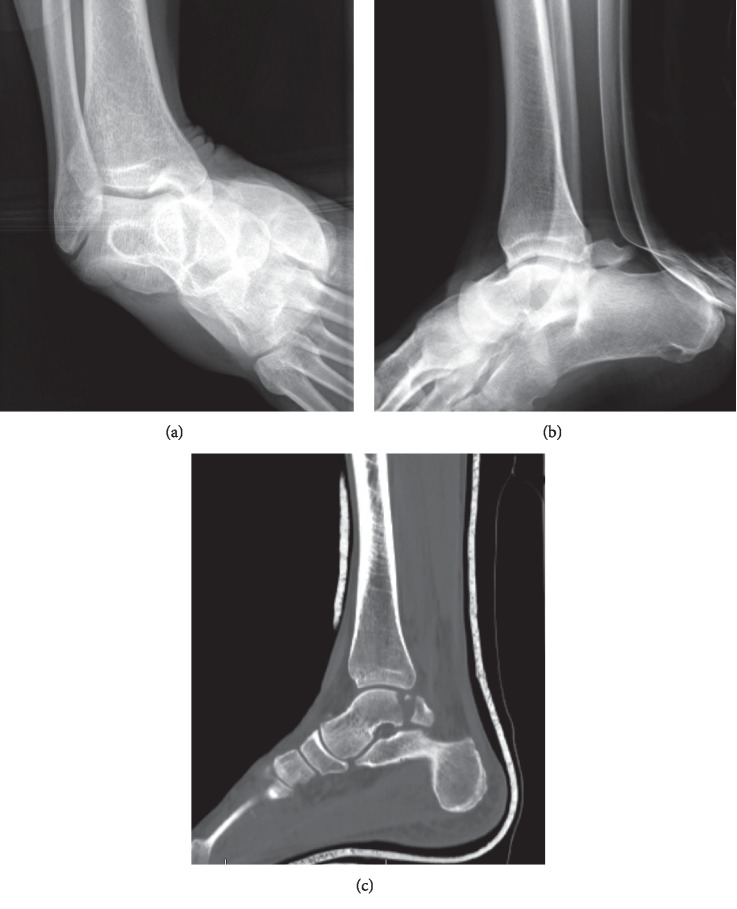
AP (a) and lateral (b) ankle X-rays demonstrating a medial subtalar fracture dislocation. The talar dome remains in normal articulation with the ankle joint, with the ankle mortise intact. Postreduction CT (c) reveals a coronally oriented, comminuted, and displaced fracture of the posterior talar dome and posterior talar process, with intraarticular extension into the subtalar joint.
